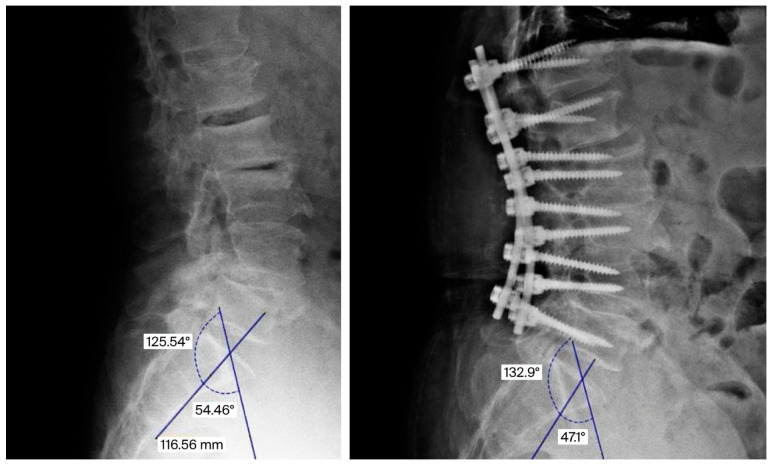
Lateral radiographs demonstrating measurement of lumbar lordosis.
